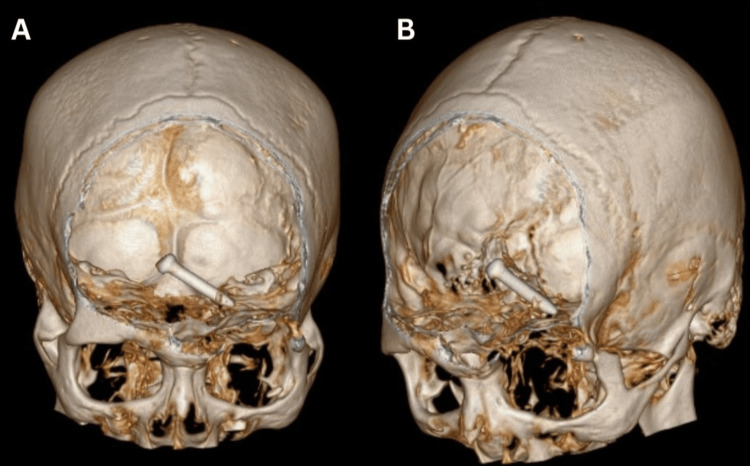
A three-dimensional reconstructed CT of the brain showing the nail penetrated in the left orbital roof and lodged in the frontal lobes.
